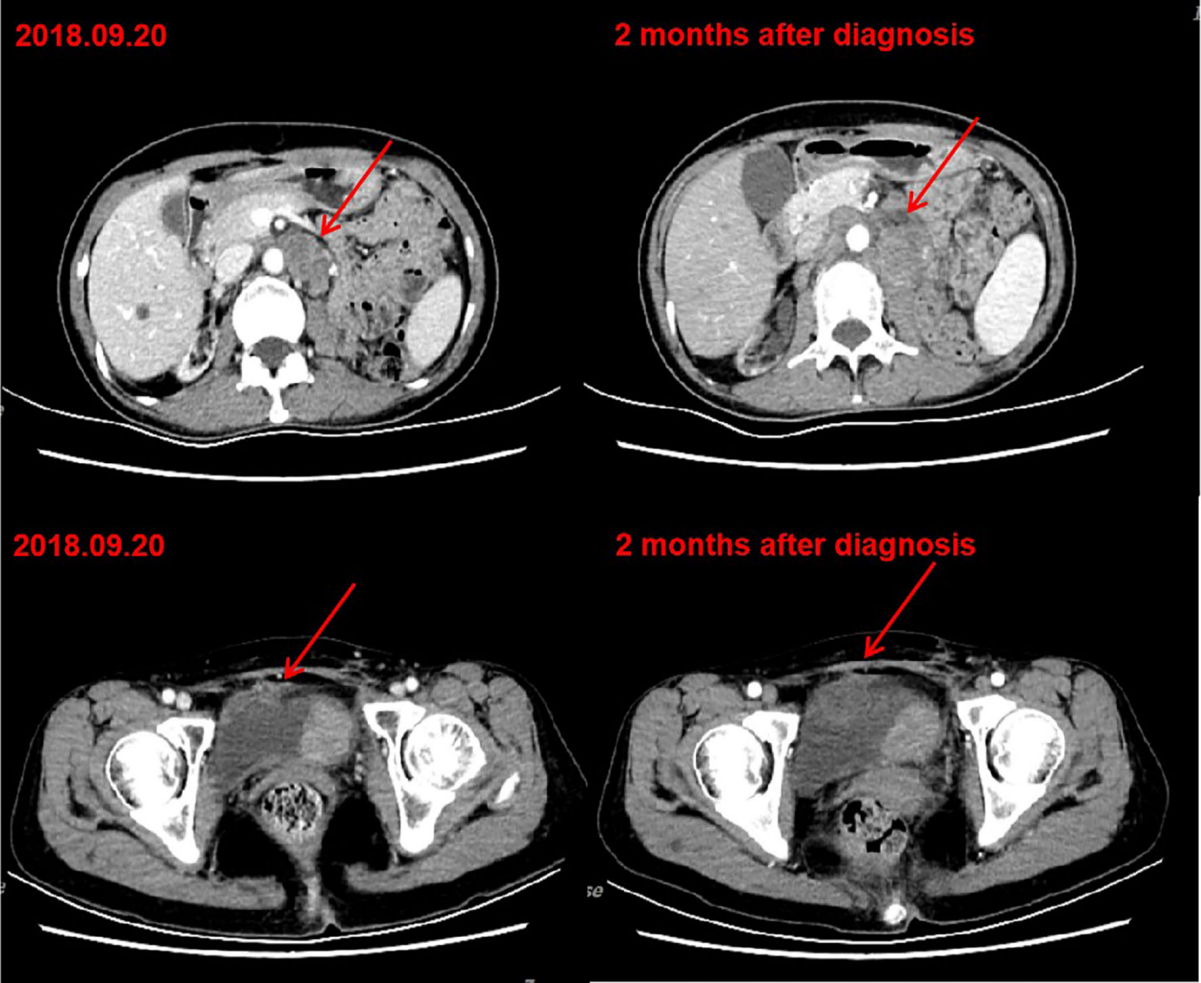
CT after chemotherapy indicated tumor progression. Left: CT of September 20, 2018. Bladder cancer with retroperitoneal and pelvic lymph node metastasis. Right: CT of November 12, 2018. After two cycles of gemcitabine chemotherapy, retroperitoneal and pelvic lesions progressed.
